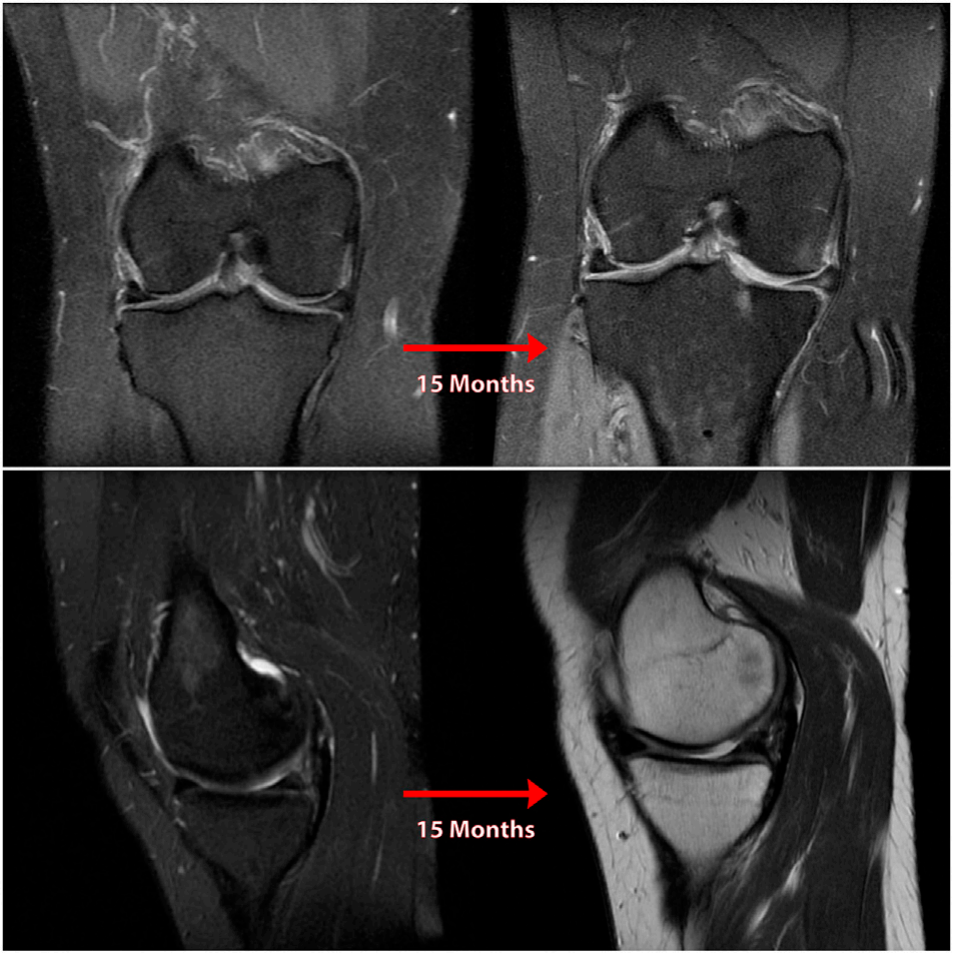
Coronal (A) and sagittal (B) MR images of nonanatomic root repair patient #2 showing progression of osteoarthritis in the medial compartment of the knee over 15 months secondary to nonanatomic medial meniscus root repair (right knee). M = medial; L = lateral; A = anterior; P = Posterior.
